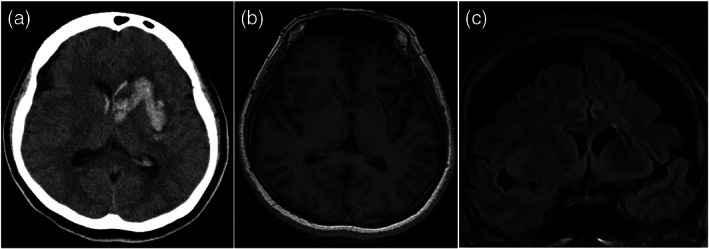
(a) The axial computed tomography image on the day of the onset of the putaminal hemorrhage. (b and c) The axial (b) and coronal (c) T1 weighted magnetic resonance imaging taken 2 years after the hemorrhage.
